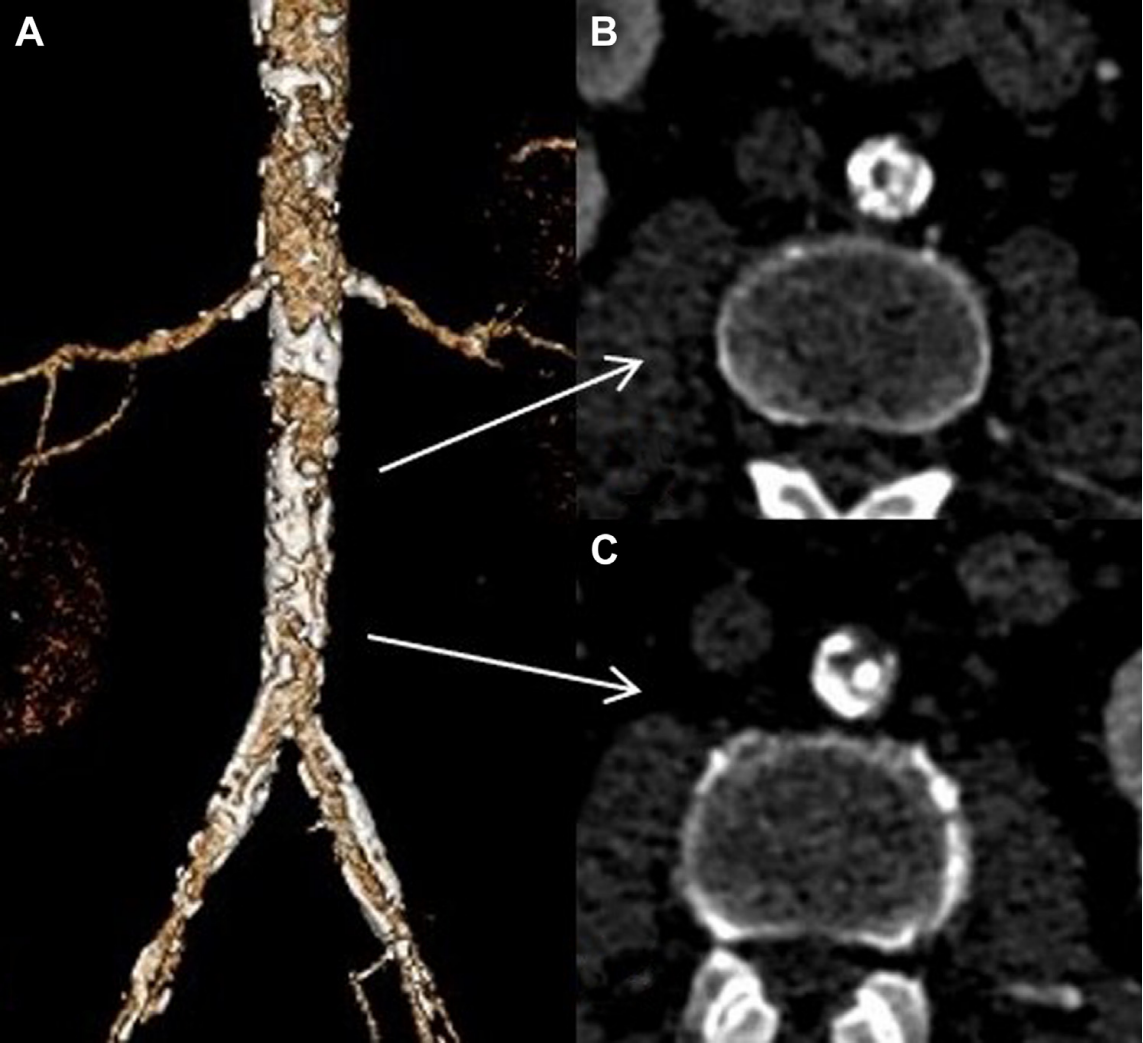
A, Three-dimensional reconstruction of preoperative computed tomography angiography (CTA) showing a “coral reef” aorta with occlusion of the mid-infrarenal aorta (B) and both common iliac arteries (C).
